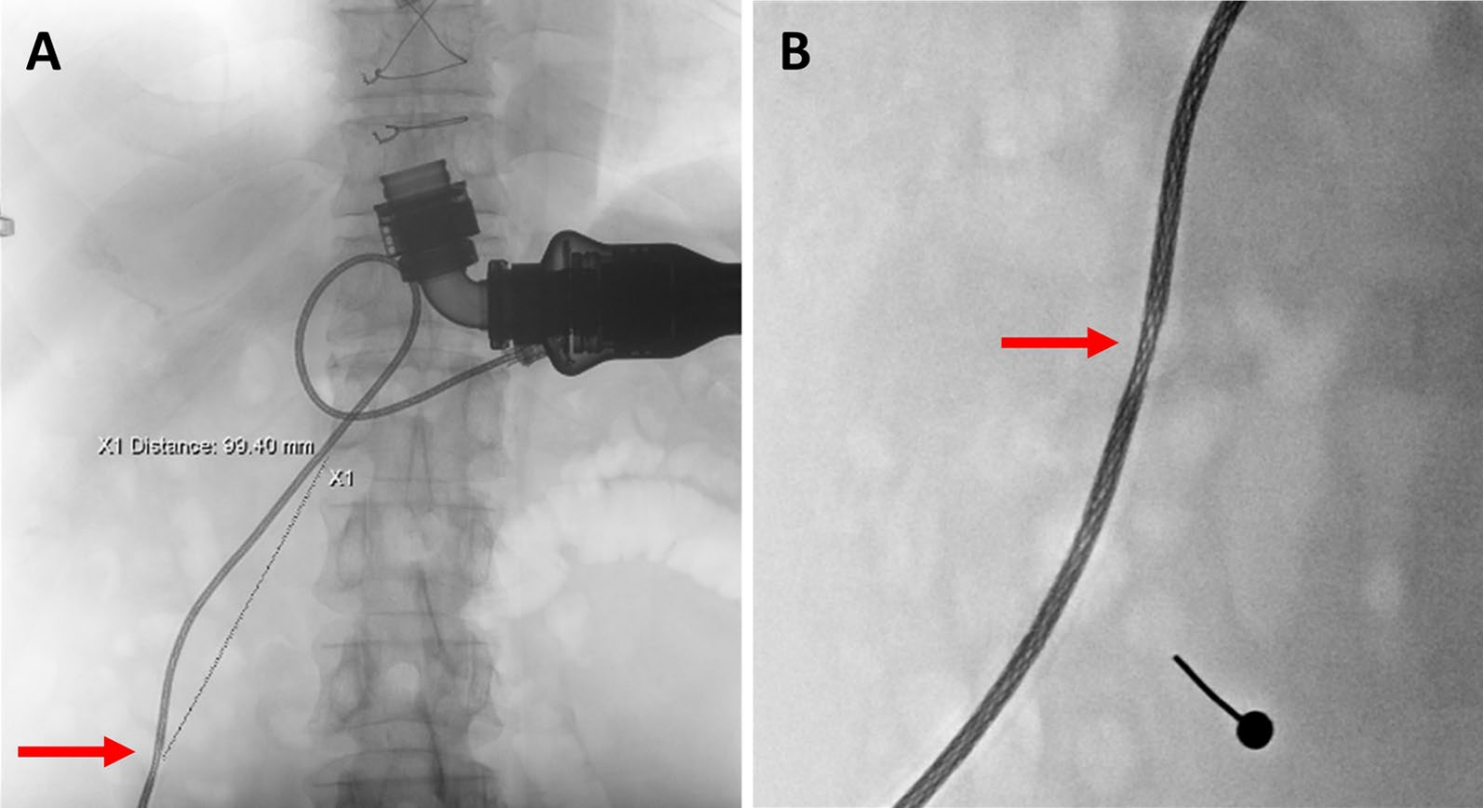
On the plain abdominal X-ray, one driveline segment (red arrows) became more radiolucent and thinner than the rest. The feature indicated the damaged part of the driveline
